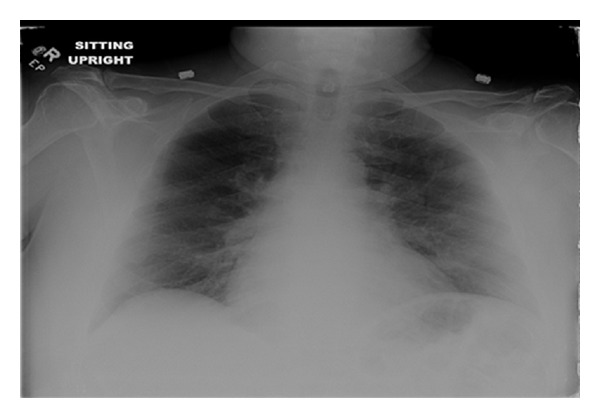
Presurgical chest radiography negative for metastasis.
